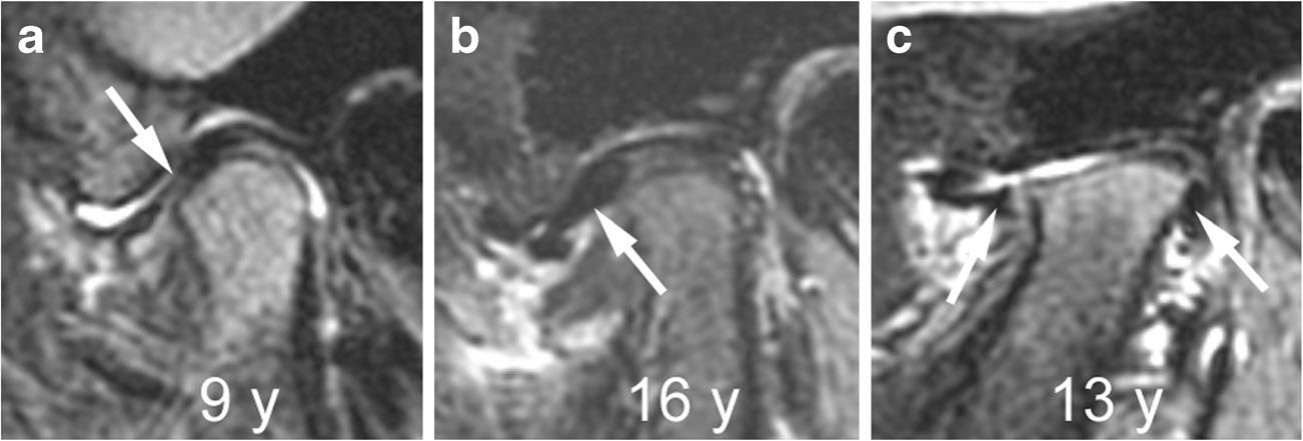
Abnormalities of articular disk. a-c Sagittal oblique fat-saturated T2-weighted fast spin echo images (TR/TE 5,400/77 ms) from different children show a flat disk (arrow) in a 9-year-old (a), an anteriorly dislocated disk (arrow) in a 16-year-old (b) and a perforated disk with peripheral remnants (arrows) seen in the anterior and posterior joint recesses in a 13-year-old (c)
